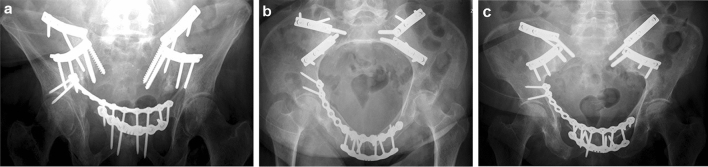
Post-operative conventional X-rays. From left to right: AP view (a), inlet view (b), outlet view (c). End result of the intervention. The SI joints posteriorly are debrided and fixed. The symphysis is double plated anteriorly, the superior plate extends over the osteotomies of the right ischio- and ilio-pubic rami and the pelvic ring is closed
